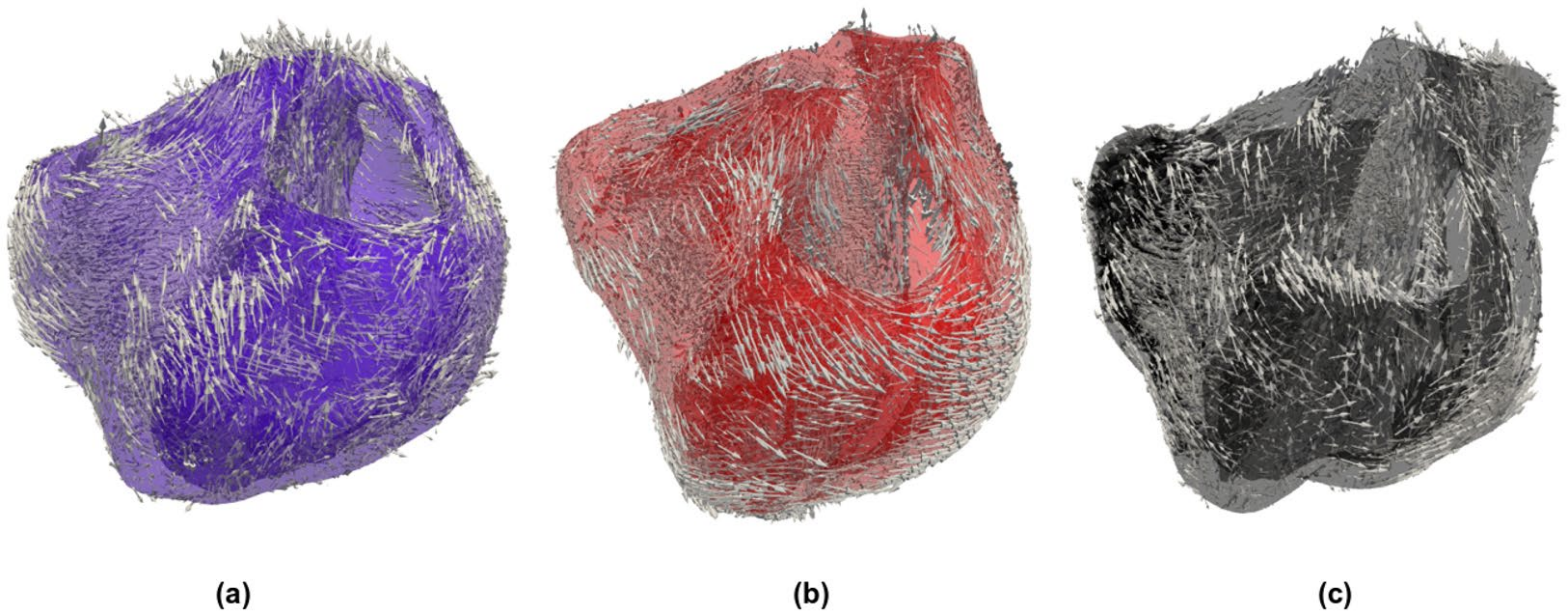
Heart geometry and fiber directions: (a) normal control (NC) heart, (b) heart-failure heart (HFC), (c) heart-failure heart treated with biomaterial injections (HFI). The geometry was reconstructed from high-resolution magnetic-resonance images (MRI), and the fiber directions were obtained from diffusion-tensor MRI.
